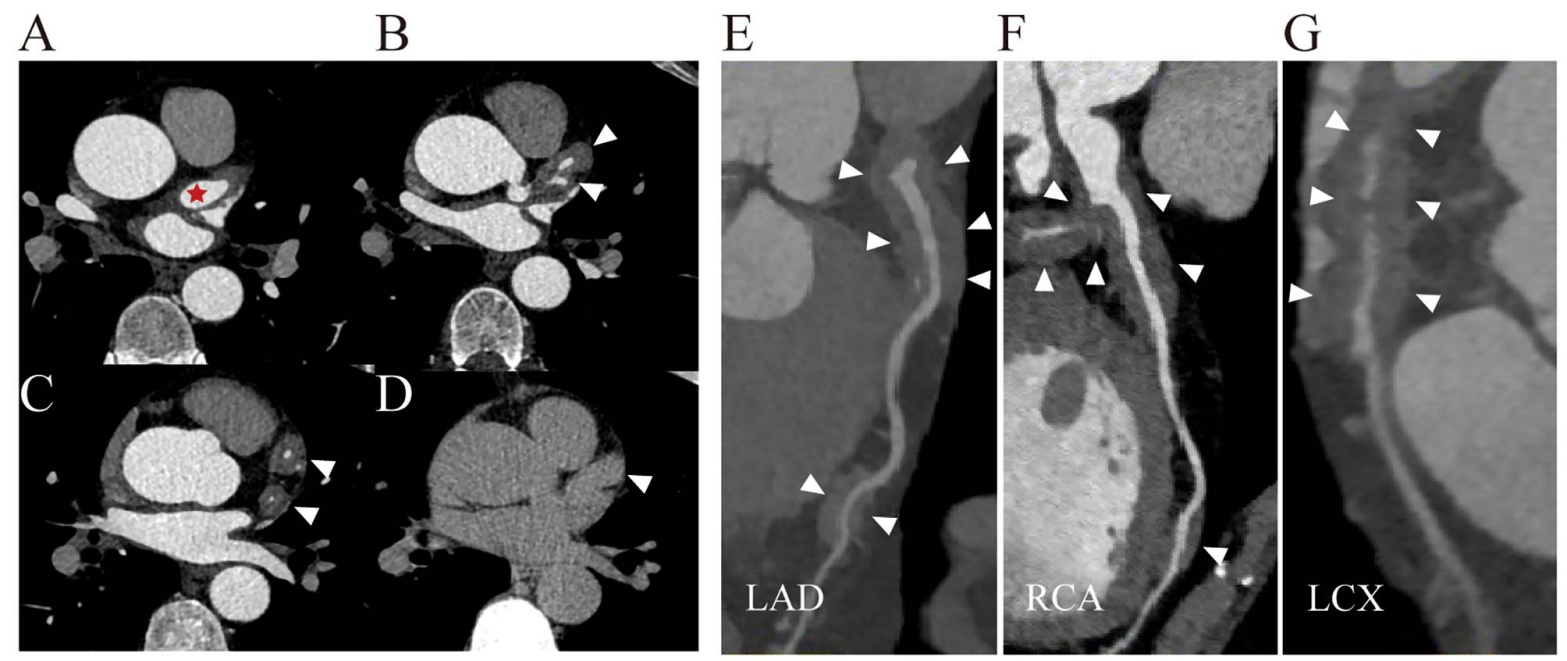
(A) showed aneurysmal dilatation of left main artery(LM), about 1.1 cm in diameter at its widest point (red asterisk). (B-D) images of the LAD and LCx showing diffuse circumferential mural thickening with associated enhancement of the wall (triangle). Curved multiplanar reformatted coronary artery CTA (E-G) demonstrate localized annular sheath-like soft tissue density shadow around LAD, RCA and Lcx (triangle)
